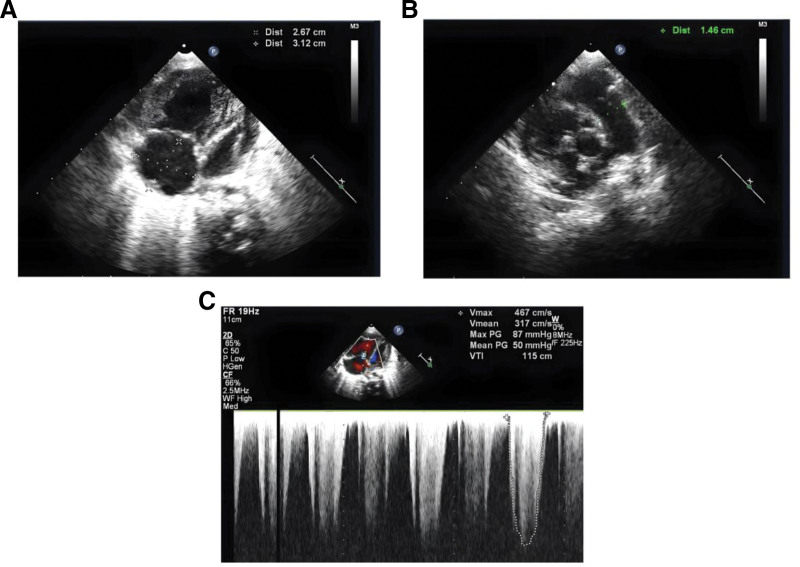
(A) The right atrium and right ventricle were enlarged, the left heart was changed under pressure, and the right ventricle wall was thickened; (B) CW Doppler evidences high-velocity tricuspid insufficiency: 4.67 cm/sec corresponding to pulmonary pressure of 87 mm Hg; (C) The inner diameter of pulmonary artery widened, 1.46 cm. C = color doppler, CF = color flow, CW = continuous wave doppler, FR = frame rate, HGen = harmonic generation, P = power doppler, PG = pressure gradient, V = velocity, VTI = velocity time integral, WF = wall filter.
